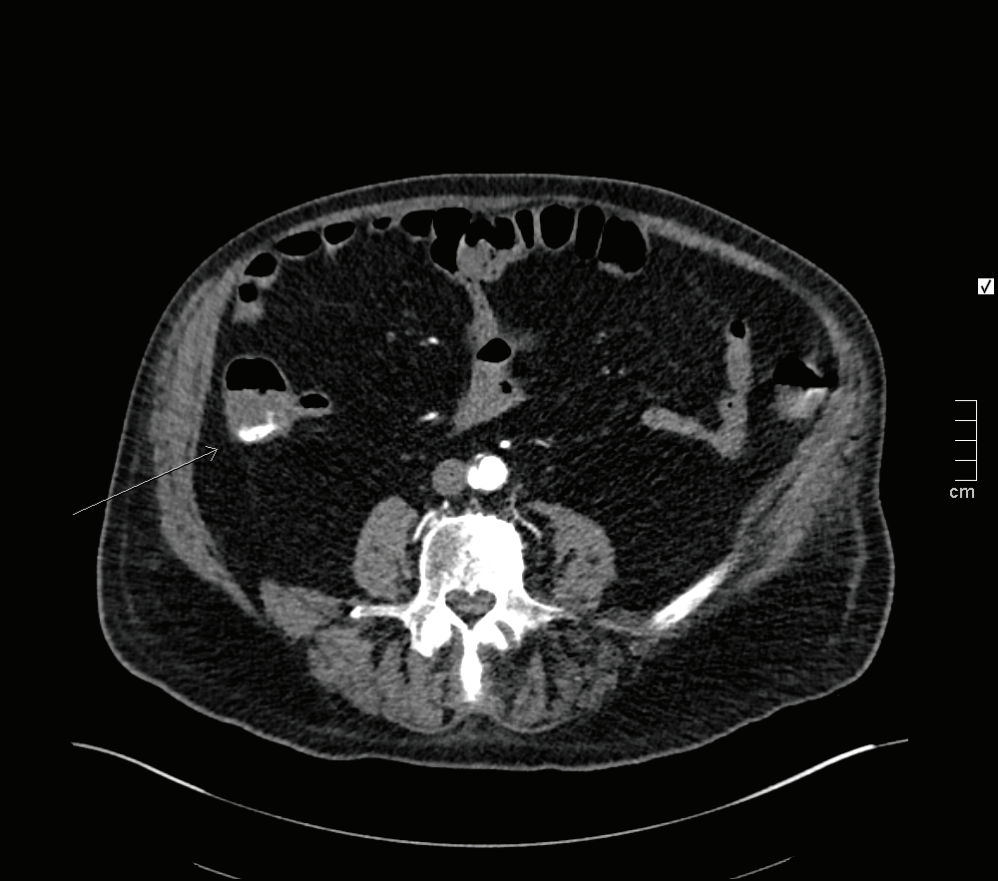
CT angiogram demonstrating active contrast extravasation in the caecum (arrow
